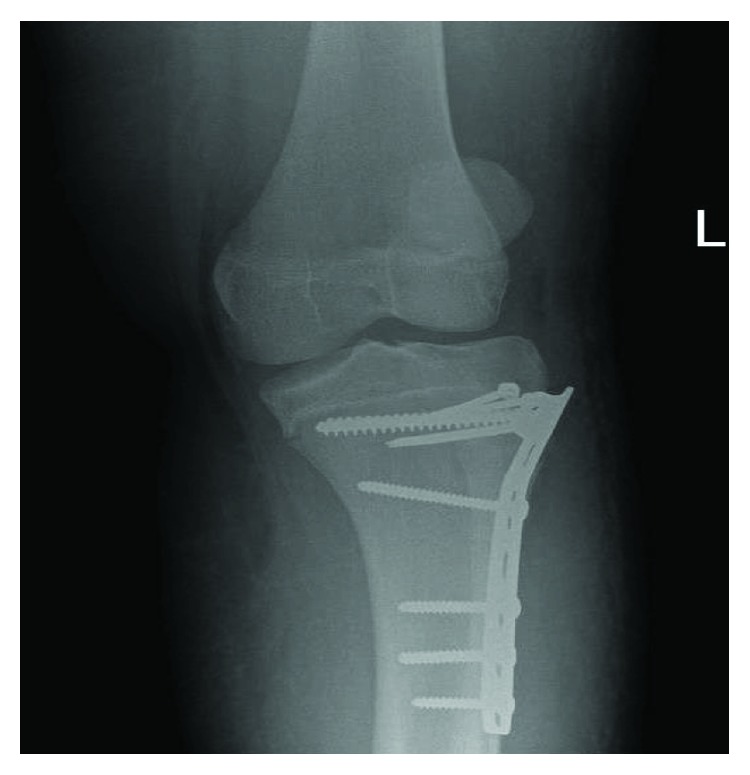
AP X-ray of the left knee 3 weeks post-op.
